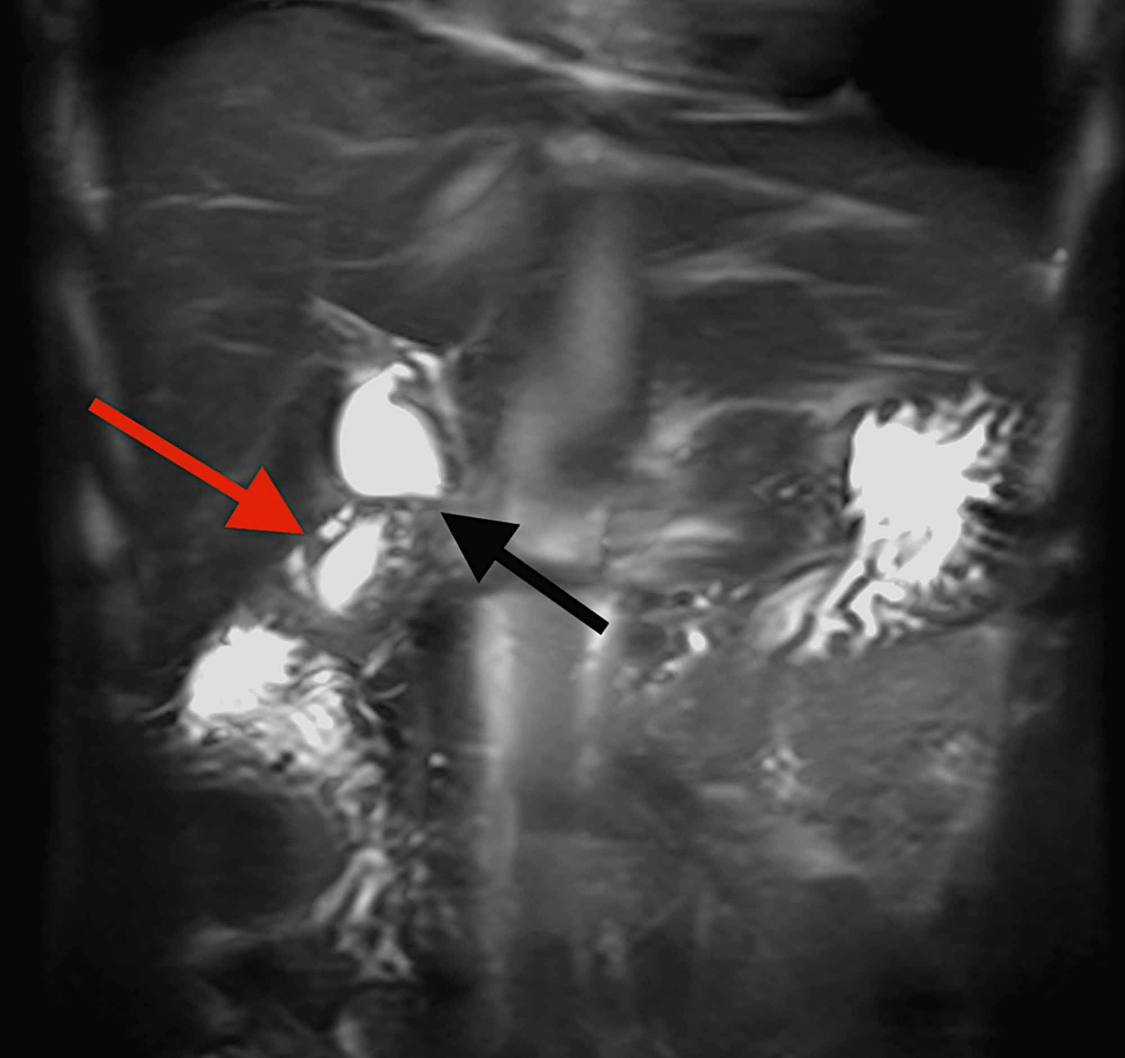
Coronal T2-weighted MRI image showing mural thickening of the gallbladder fundus and body with fluid-filled intramural diverticula (red arrow) and an hourglass configuration (black arrow) consistent with gallbladder adenomyomatosis (segmental type).
